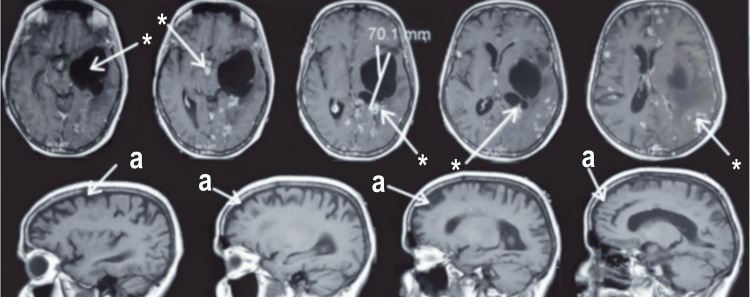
Brain MRI, axial section with T1-weighted sequence post-gadolinium injection, and sagittal T1-weighted sequence of a 47-year-old female patient with neurosarcoidosis associated with a left temporal 70 mm long-axis liquid lesion *: Diffuse micro and macronodular thickening of the meninges above and below the tentorium with a predominantly hemispheric involvement in the left periventricular and pericallosal areas, hyperintense on FLAIR, enhancing significantly after contrast administration. a: Pachymeningitidis FLAIR: fluid-attenuated inversion recovery
